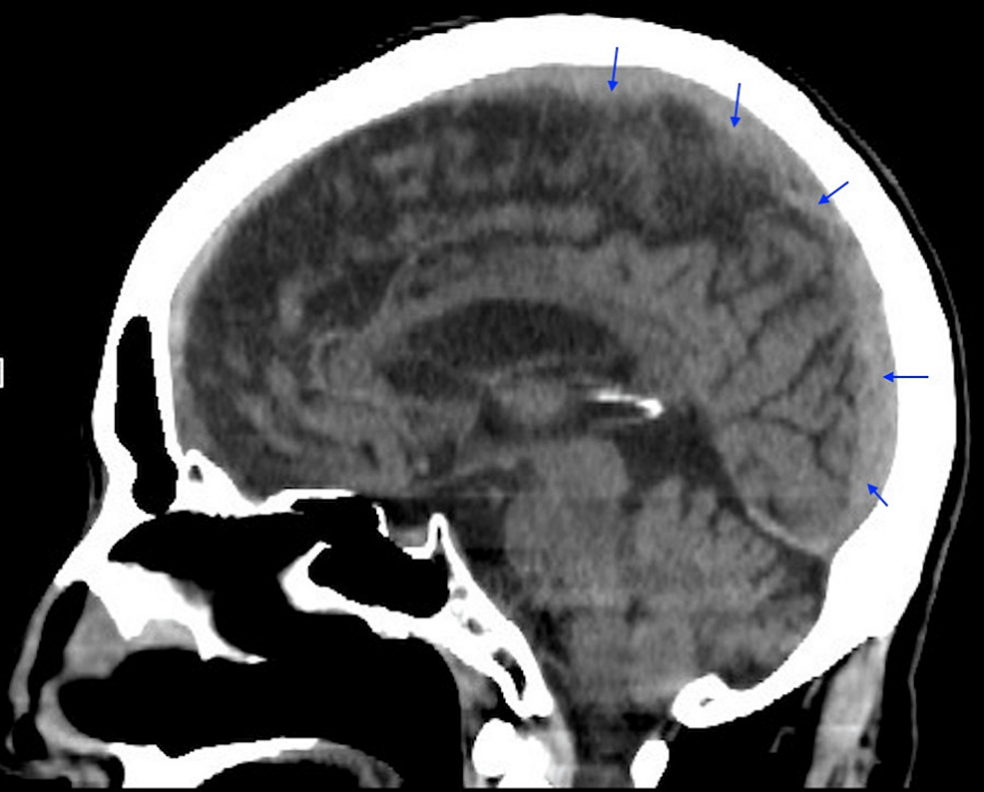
Sagittal view non-contrast brain computed tomography The scan reveals an ill-defined acute-on-chronic left subdural hematoma above the parietal lobe region (arrows).
